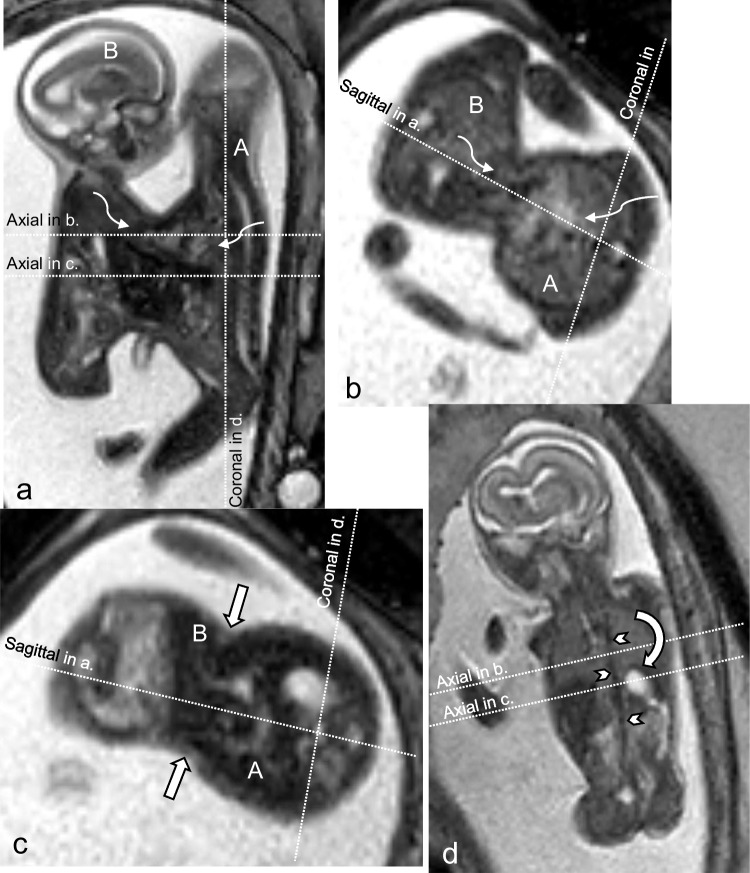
Fetal MRI of thoracopagus at gestational age 20 weeks, 4 days, demonstrating imaging planes. *Dashed lines* are labeled to indicate the approximate orthogonal imaging planes depicted in the other figure parts. a BTFE image sagittal to the ventrally conjoined torsos of the twins. This imaging plane is also sagittal to the brain of twin B. Twin A’s head is turned out of the imaging plane. Dedicated imaging of the twins’ brains required individualized imaging planes (*not shown*). Much of the shared heart (*between thin curvy white arrows*) is positioned within the chest of twin A, a feature that helps to differentiate the twins. b BTFE image axial to the shared chest. Axial plane of imaging redemonstrates the shared heart eccentrically positioned within twin A (*between thin curvy white arrows*). c BTFE image axial to the asymmetric shared abdomen. Twin A’s abdomen is larger and contains more of the shared liver (*between white arrows*) than twin B’s abdomen. d T2 SSH coronal to twin A. Left-sided aorta (*white arrowheads*) and stomach (*curved white arrow*) demonstrated. *BTFE*, balanced turbo-field echo; *SSH TSE*, single-shot turbo spin-echo
